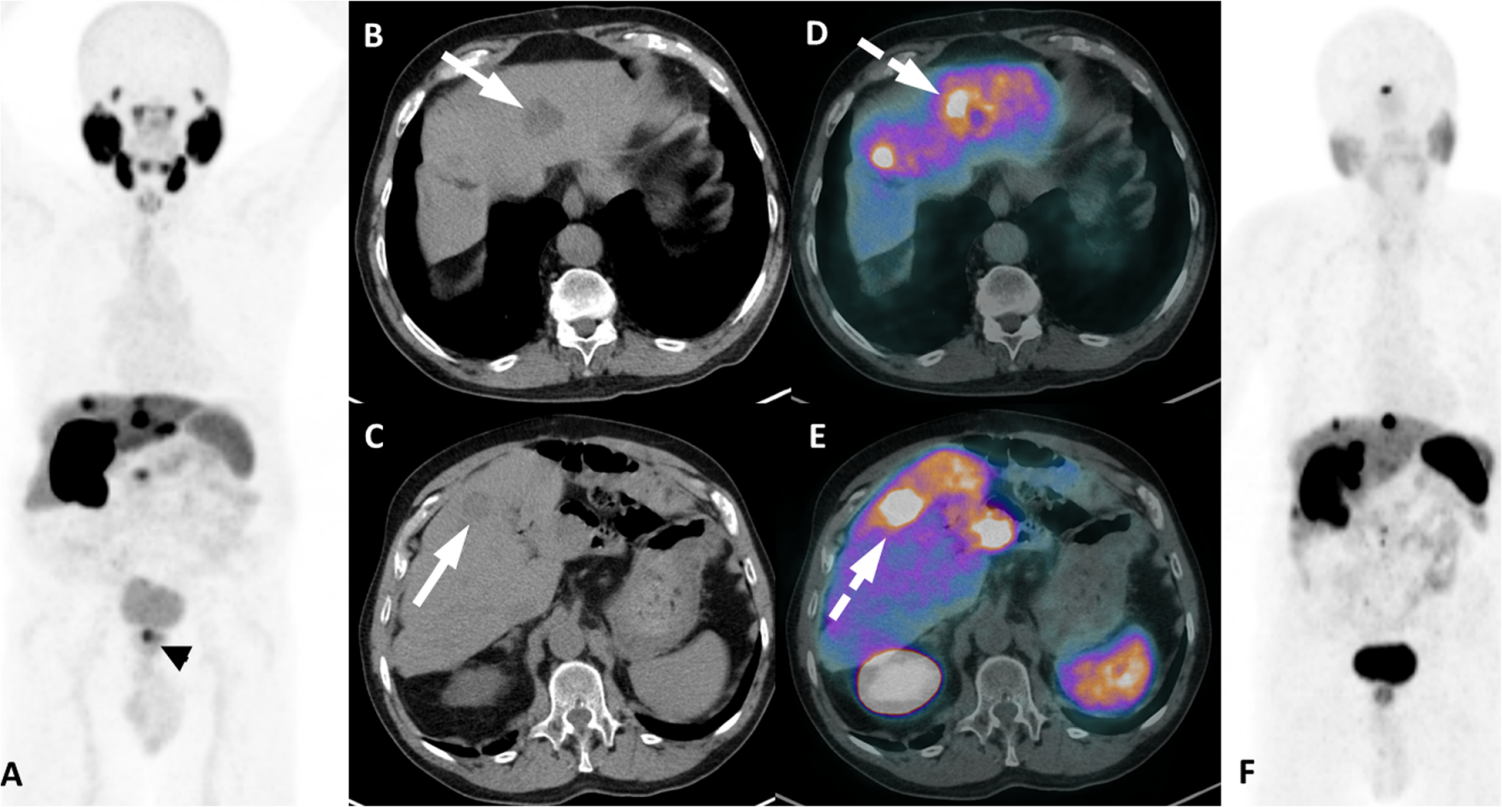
Neuroendocrine liver metastases in ^68^Ga-PSMA and ^68^Ga-DOTATATE PET. ^68^Ga-PSMA PET MIP (a), axial CT (b, c) and axial PET/CT (d, e) show a high uptake (dashed arrows) in metastatic liver lesions of neuroendocrine tumor (arrows). Note also the primary prostate tumor (arrowhead in A). ^68^Ga-DOTATATE PET MIP (f) 2 years before showing that these hepatic lesions had also high somatostatin receptors expression
